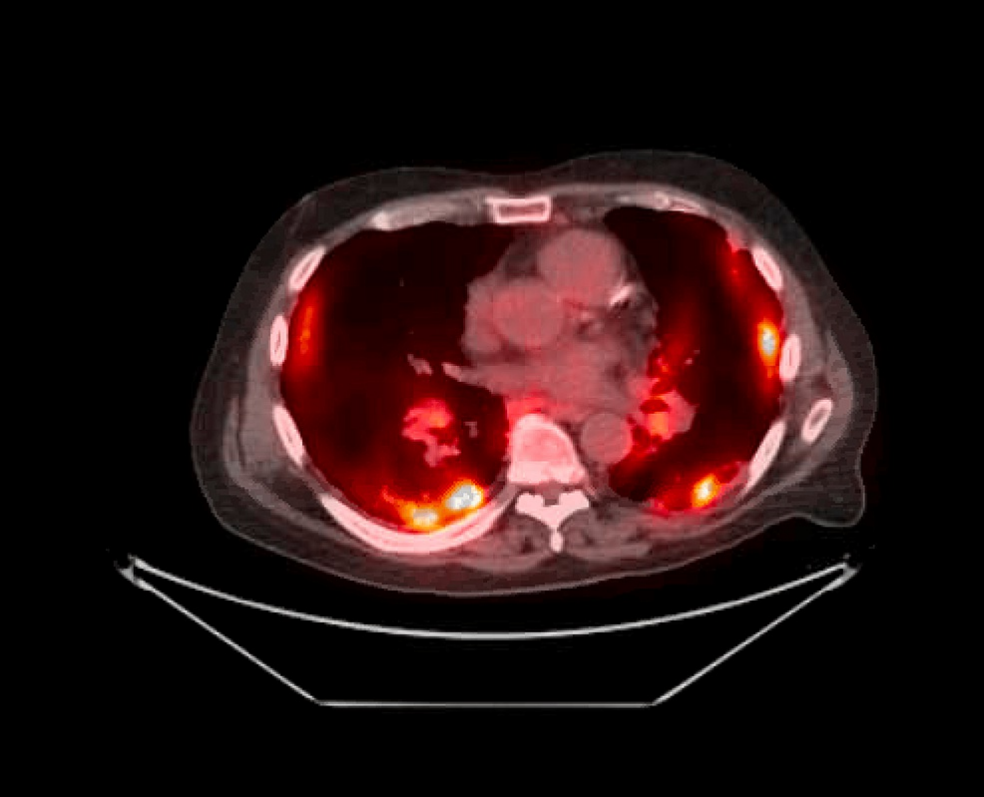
Repeat PET-CT scan The image is showing a mixed response to chemotherapy with hyper-metabolic activity bilaterally in all areas with reduced activity in the primary left lower lung base. PET-CT: Positron emission tomography-computed tomography
